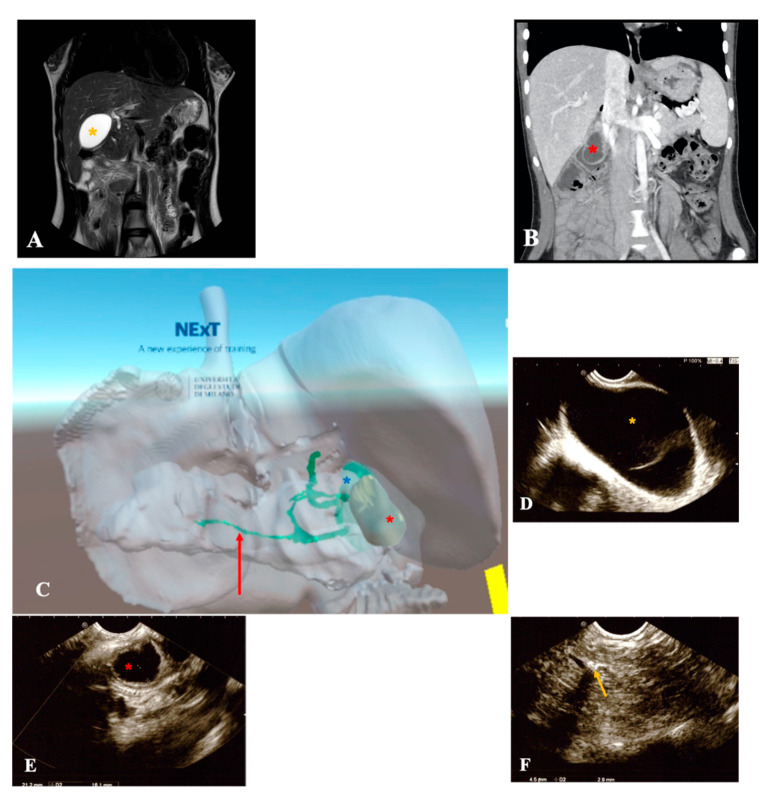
Patient n. 4 underwent surgery in the neonatal period for duodenal atresia. Years later, she developed symptomatic pancreato-biliary tree stones with cholelithiasis and choledocholithiasis. (A,B): Cholangio MRI showed a dilated gallbladder (yellow asterisk, (A)) with stones in the infundibulum and the choledochal channel near a cystic dilatation. (D–F): EUS confirmed the presence of a dilated gallbladder (yellow asterisk, (D)). We also identified a stone in the minor pancreatic duct (yellow arrow, (F)). (C): On the posterior view of the 3D reconstructions, the choledochal channel appears to end in the upper duodenal stump with a dilatation (blue asterisk in (C)). The pancreatic duct (red arrow in (C) ends in the lower duodenal stump. The upper duodenal stump also appears on endoscopic US as a blind-ending pouch (red asterisk in (C,E)).
